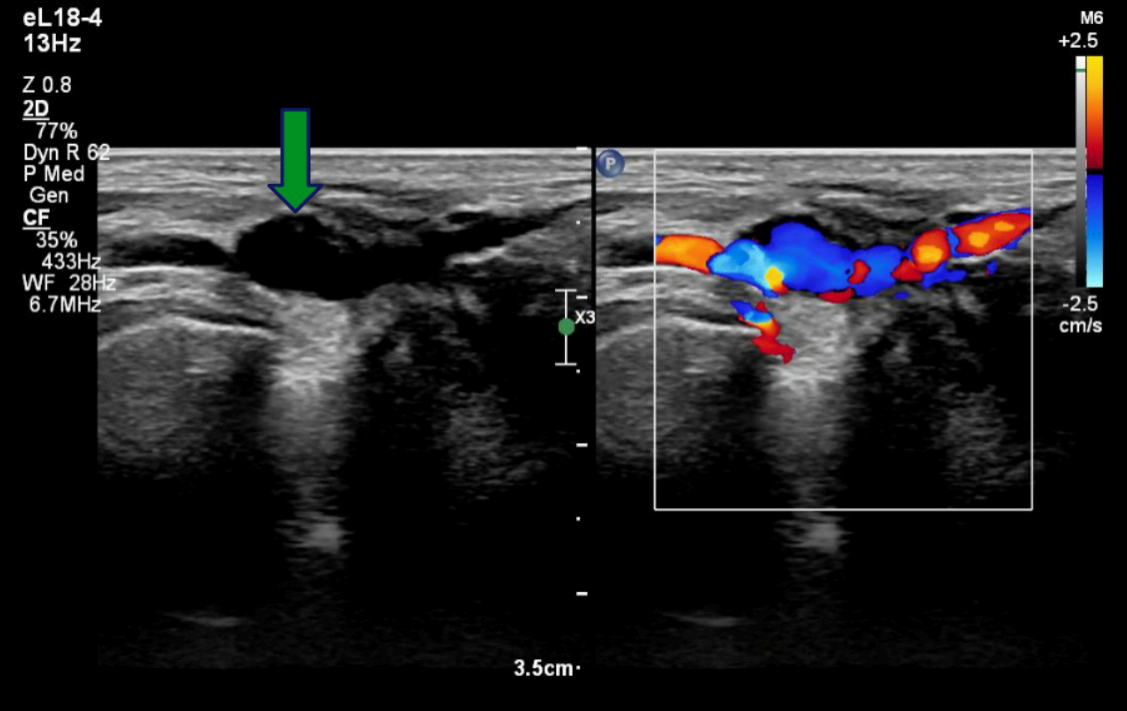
US image (left), showing aneurysmal dilation (at tip of arrow) in the center of the image. Doppler US (right), showing turbulent flow in the region of the aneurysm.
